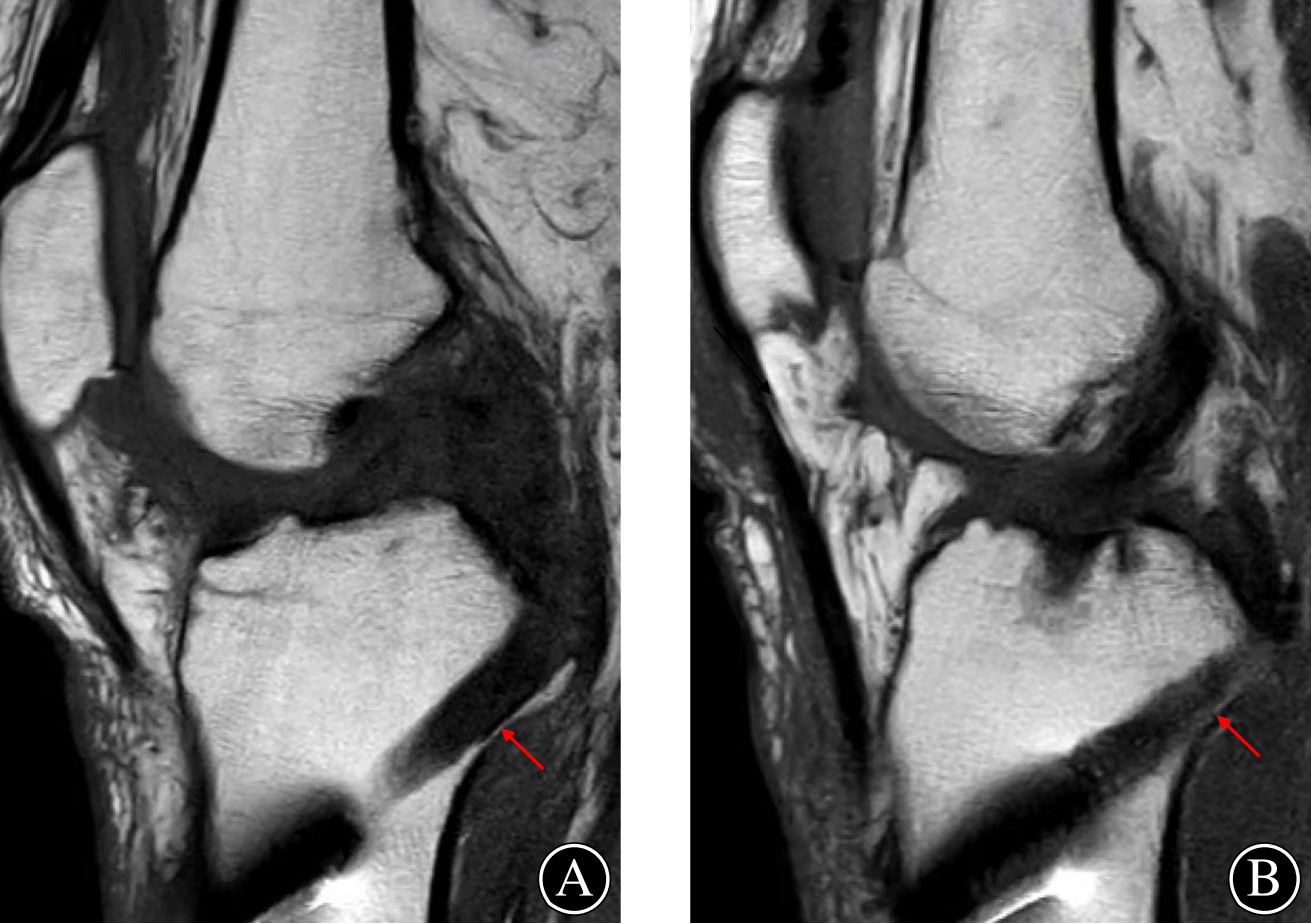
Two cases of PCL tibial tunnels on MRI images: red arrows showed that the posterior wall of tibial tunnel was very close to the posterior tibial cortex.
